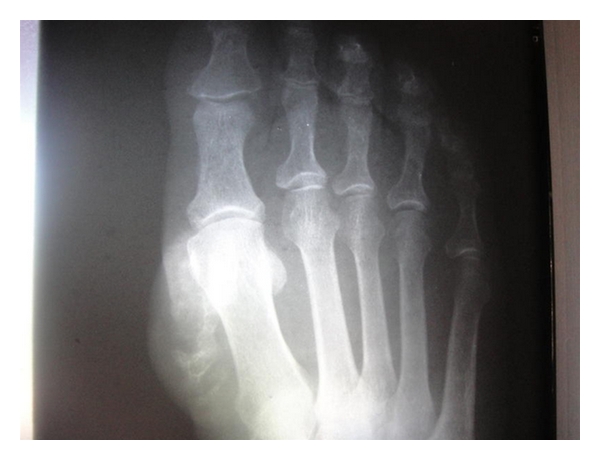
Radiograph showing soft tissue mass with calcification.
